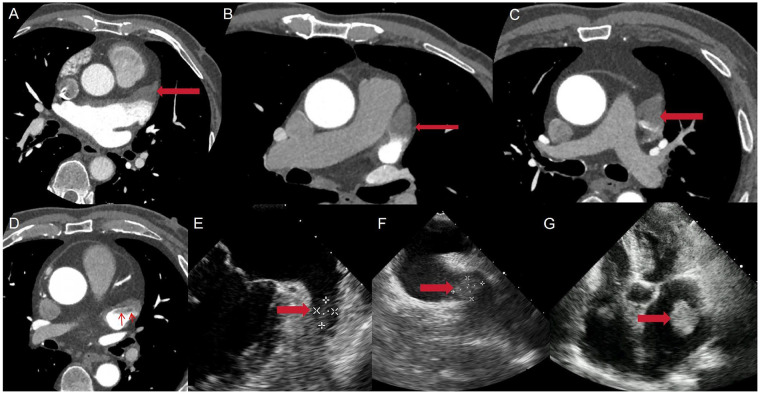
Imaging findings of left atrial appendage (LAA) thrombi. (A) CTA of the left atrium showing a thrombus localized to the tip of the LAA and occupying less than half of the interior of the LAA. (B) CTA image showing a thrombus occupying more than half of the interior of the LAA but not extending beyond the LAA orifice. (C) CTA image demonstrating complete filling of the LAA by the thrombus. (D) Image of the same patient as in Panel C, demonstrating a LAA thrombus that extends beyond the LAA orifice and protrudes into the LA. (E) TEE reveals thrombus formation within the LAA tip that occupies less than half of the interior of the LAA. (F) TEE reveals a thrombus that occupies more than half of the interior of the LAA but not extending beyond the LAA orifice. (G) TTE shows a thrombus within the LAA extending into the LA. (The thrombus is indicated by a red arrow in each panel).
